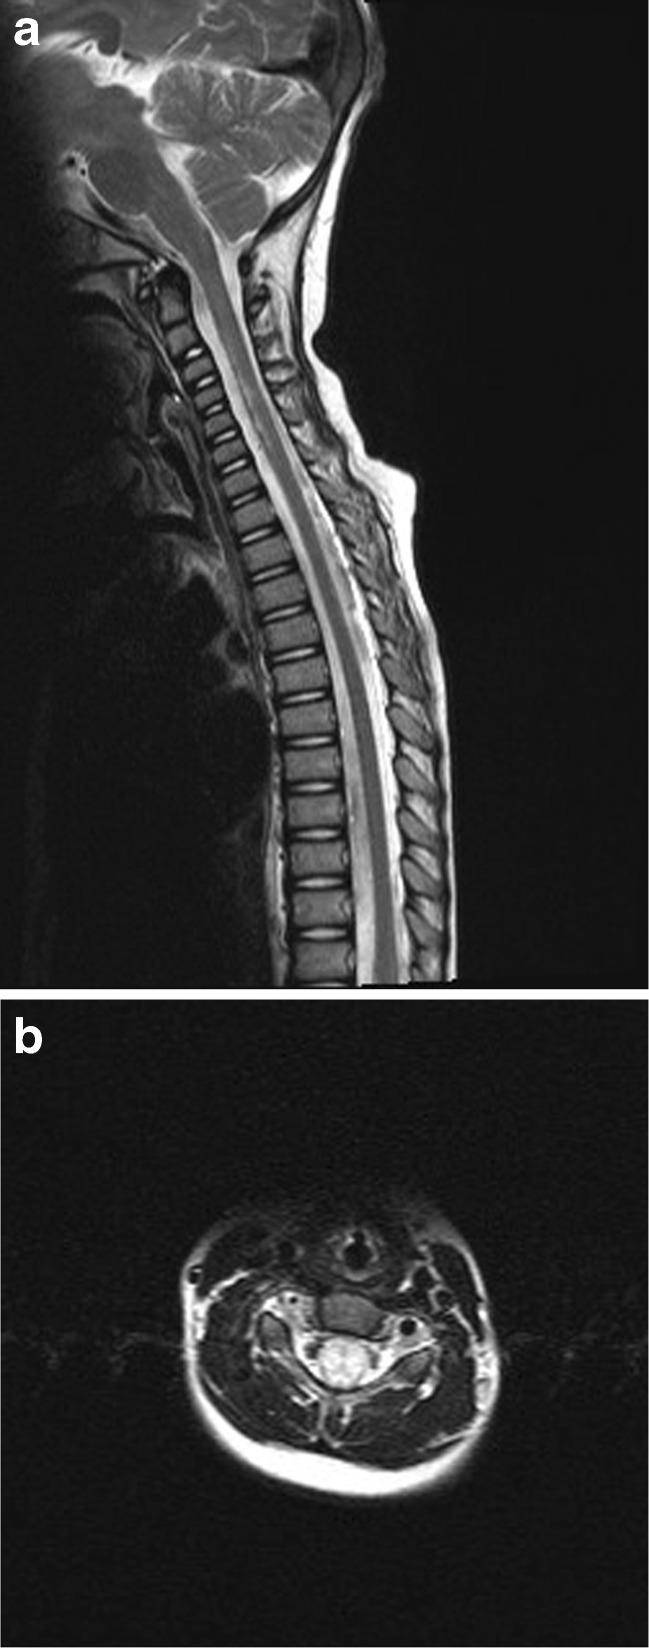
Follow-up MRI at 6 weeks confirms interval volume loss and established myelomalacia on sagittal (a) and axial (b) T2 imaging
